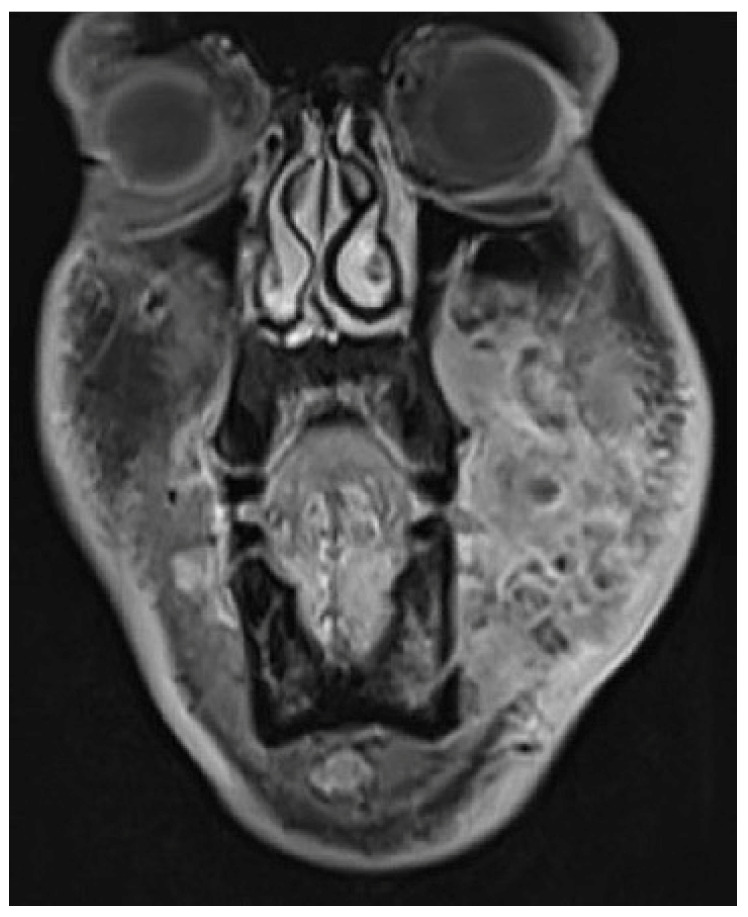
Case 5—Preoperative staging T2W magnetic resonance imaging (coronal view).
